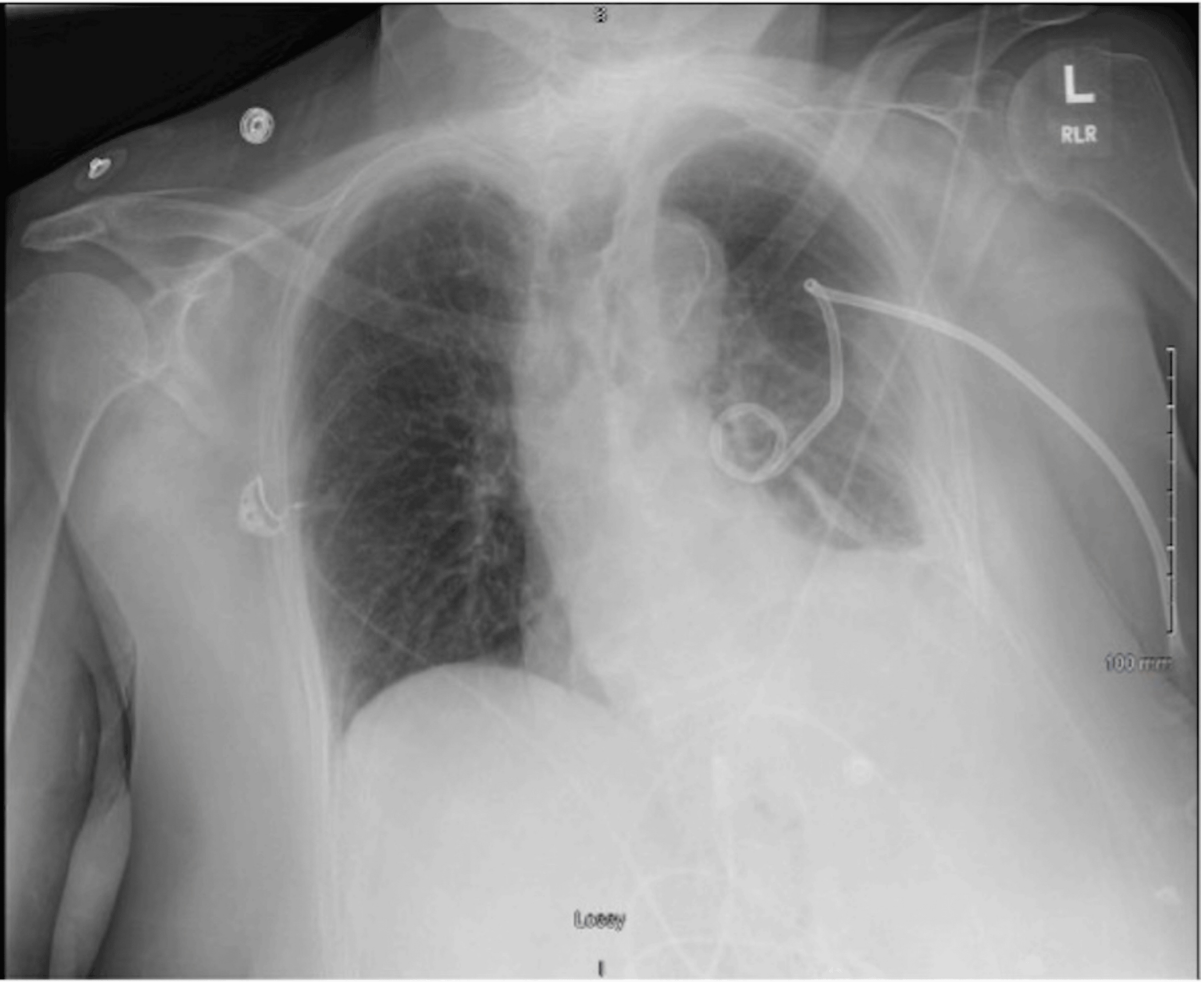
An anteroposterior (AP) chest x-ray. The image shows interval improvement of left hemothorax, with a significant reduction in the opacification of the left hemithorax compared to prior imaging, indicating decreased pleural fluid accumulation. Improved lung aeration is noted, with the left lung demonstrating increased aeration with visible expansion of lung fields, suggesting effective re-expansion of the lung following intrapleural administration of tPA and DNase. The left-sided chest tube remains in situ, continuing to facilitate drainage of the pleural space.
